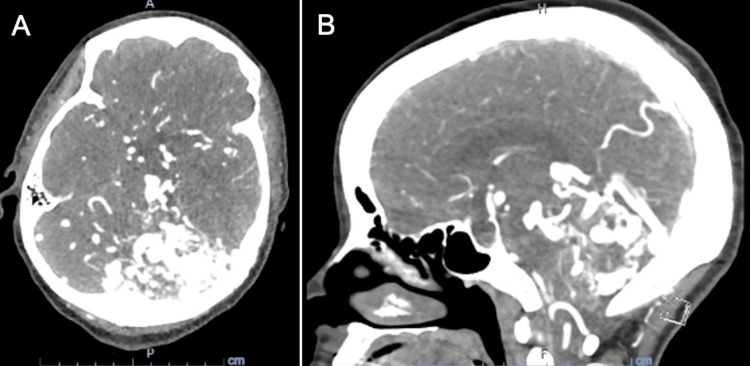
CT angiogram of the arteriovenous malformation (transverse (A) and sagittal (B) views) showing multiple arterial feeders seen from the left posterior inferior cerebellar artery and left superior cerebellar artery with dilation of the vertebral basilar system and bilateral internal carotid arteries, particularly on the left.
